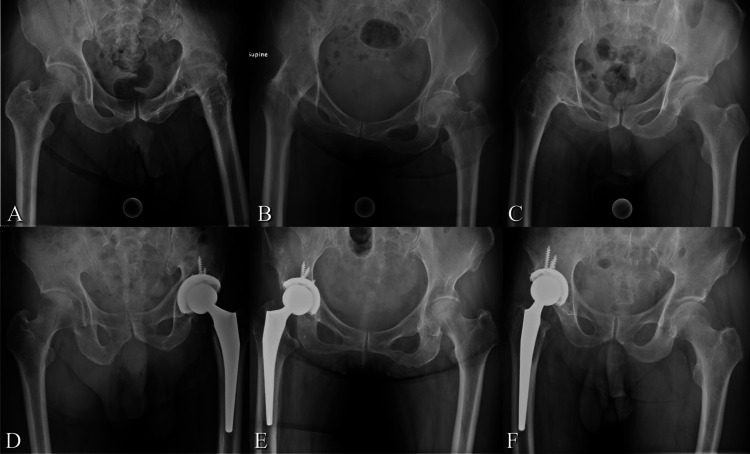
Perioperative hip joint radiographs. A, B, C: Preoperative hip radiographs of Cases 1, 2, and 3. D, E, F: Postoperative hip radiographs of Cases 1, 2, and 3. Case 1: A 65-year-old man; Case 2: A 73-year-old man; Case 3: A 75-year-old woman
